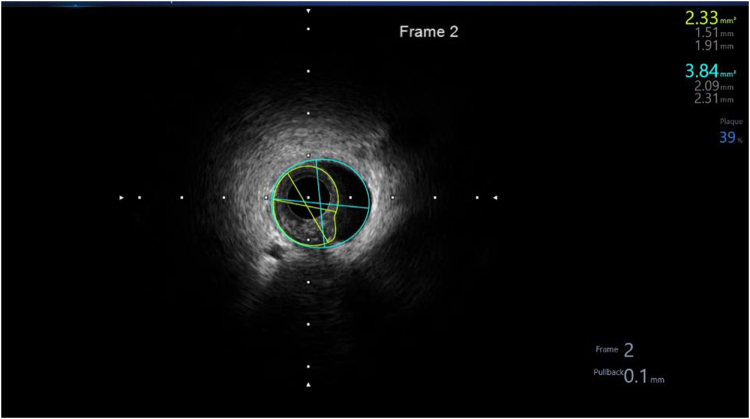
IVUS cross-sectional measurement of true lumen, false lumen, and hematoma. IVUS, intravascular ultrasound.
